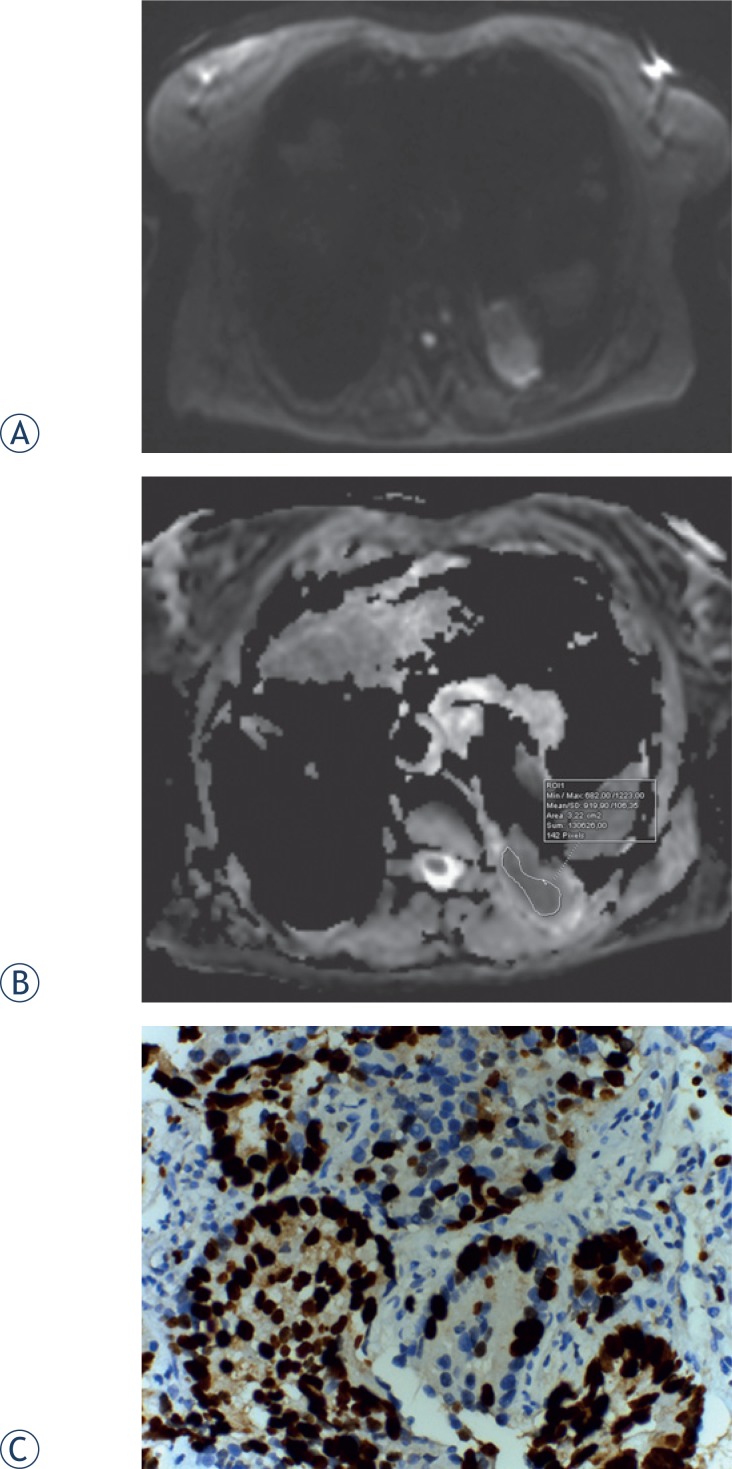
Diffusion-weighted (DW)-MRI, apparent diffusion coefficient (ADC) map of a 62-year-old female with adenocarcinoma. (A) Tumour shows heterogeneously high signal intensity on DW-MRI, for which the b value is 800 s/mm^2^. (B) On the ADC map, the tumour demonstrates heterogeneous diffusion restriction. (C) Proli ferative index 95% in glandular epithelium (Ki-67X400).
